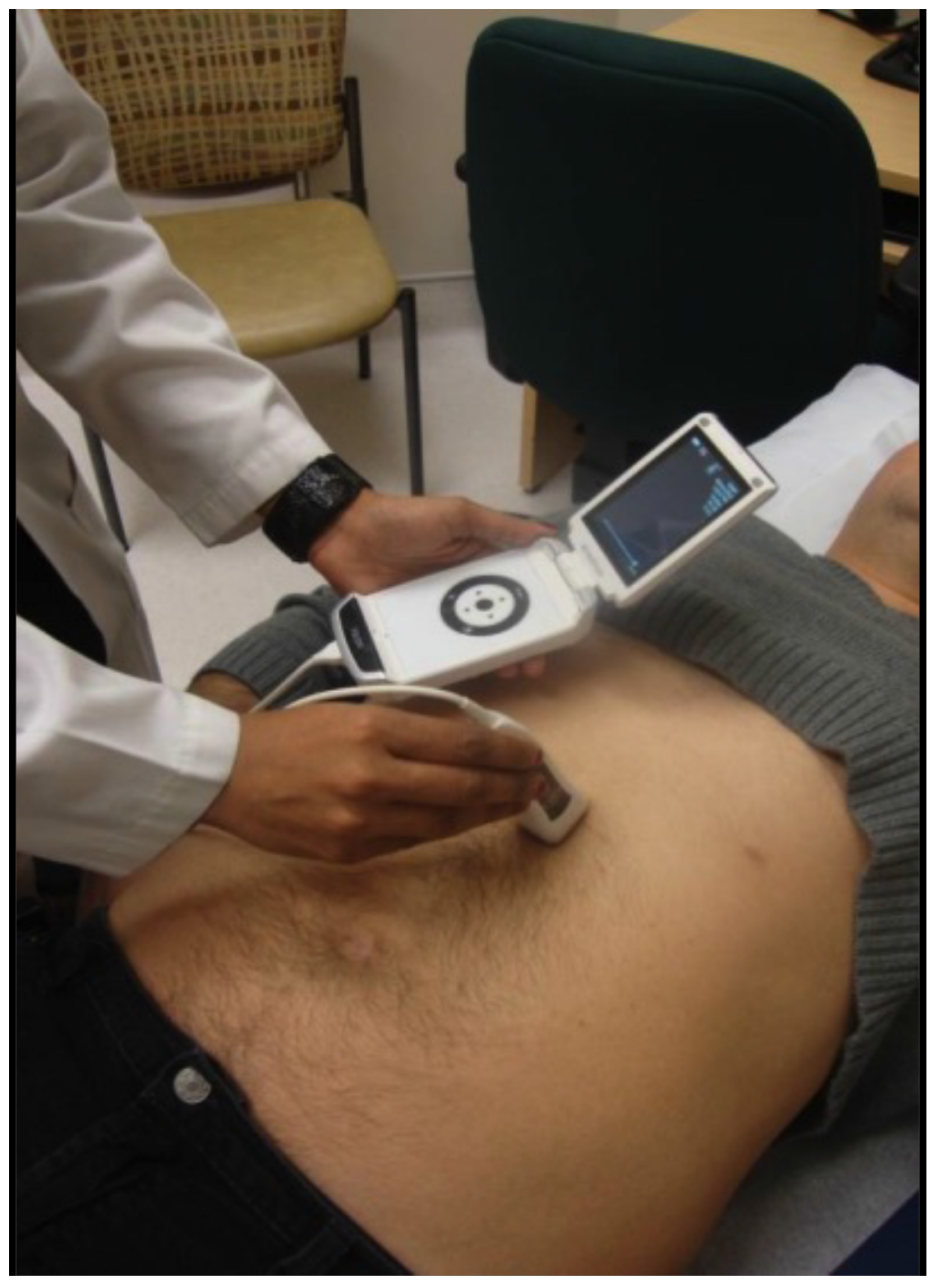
Hand-held bedside ultrasound device (Mini Echo).
